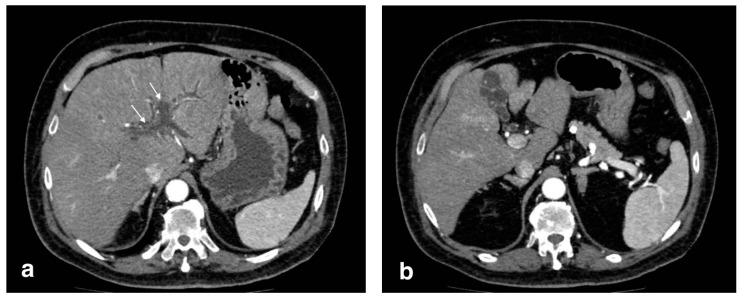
Pylephlebitis in cholecystitis exacerbation: (a) massive thrombosis of the portal bifurcation with hyperdensity of portal walls due to pylephlebitis (white arrows) and inhomogeneous attenuation of liver parenchyma in arterial CT scan due to vascular occlusion; (b) axial scan: irregular thickening of cholecystic walls with contrast enhancement of liver parenchyma due to pericholecystic edema.
